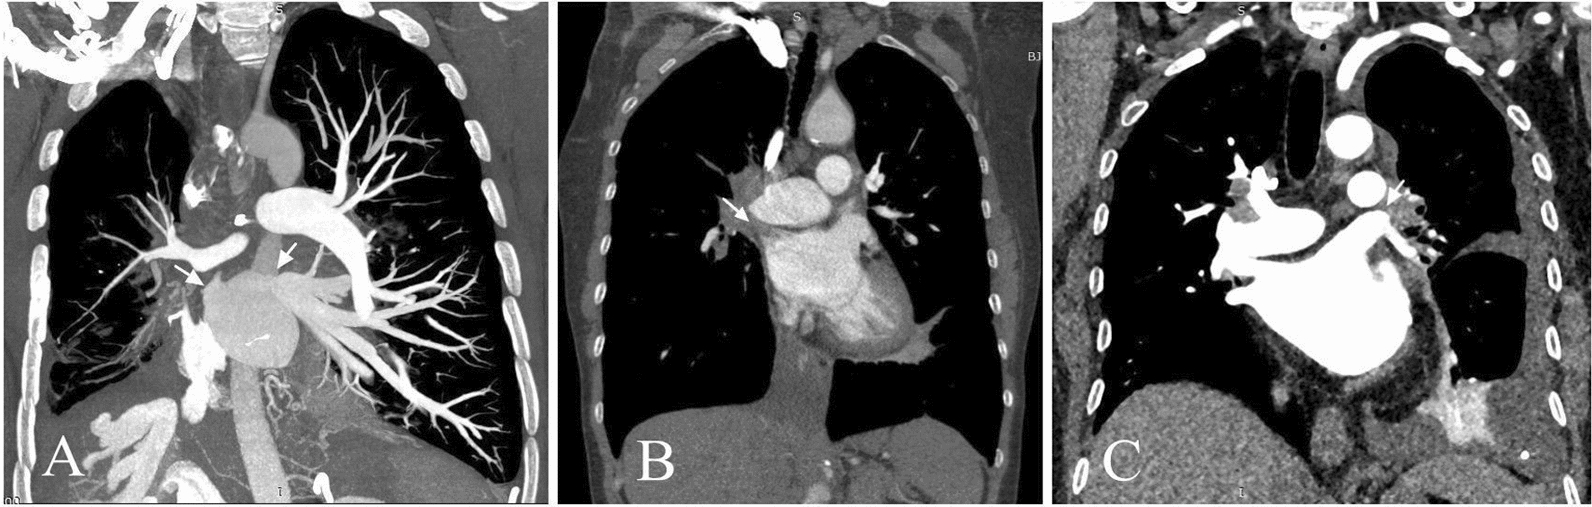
Coronal reformatted three-dimensional reconstructions images of computed tomography pulmonary angiography. Panel A shows right pulmonary vein and left superior pulmonary vein occlusion (arrow, case 2). Panel B shows right superior pulmonary vein occlusion (arrow, case 3). Panel C shows a main branch of left superior pulmonary vein occlusion (arrow, case 6)
